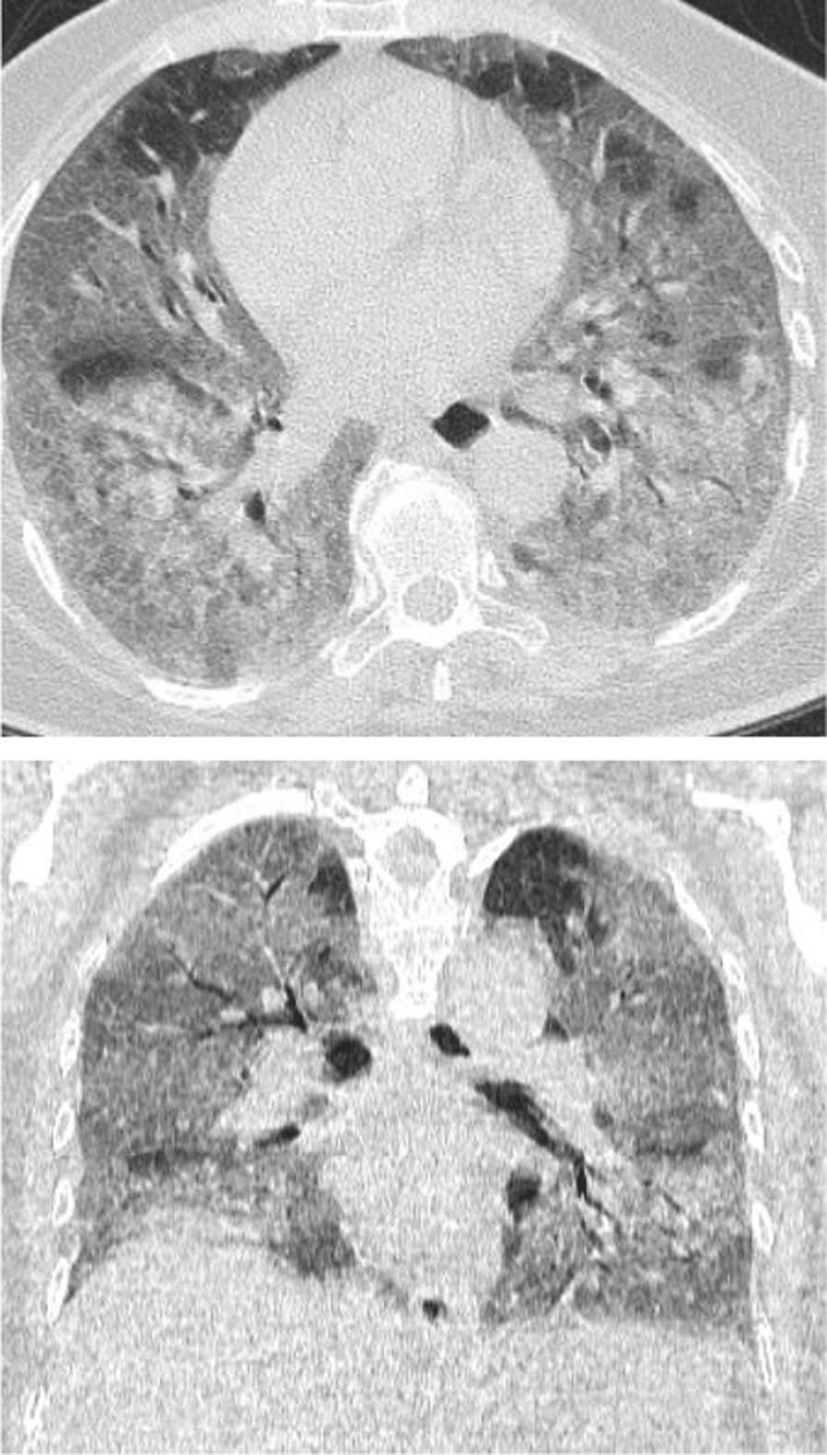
Female patient aged 71 years, with fever, dyspnea and neurological manifestations. Diffuse central and peripheral ground glass infiltration mounting to whitening out of the lung parenchyma. The CT severity score was 23
